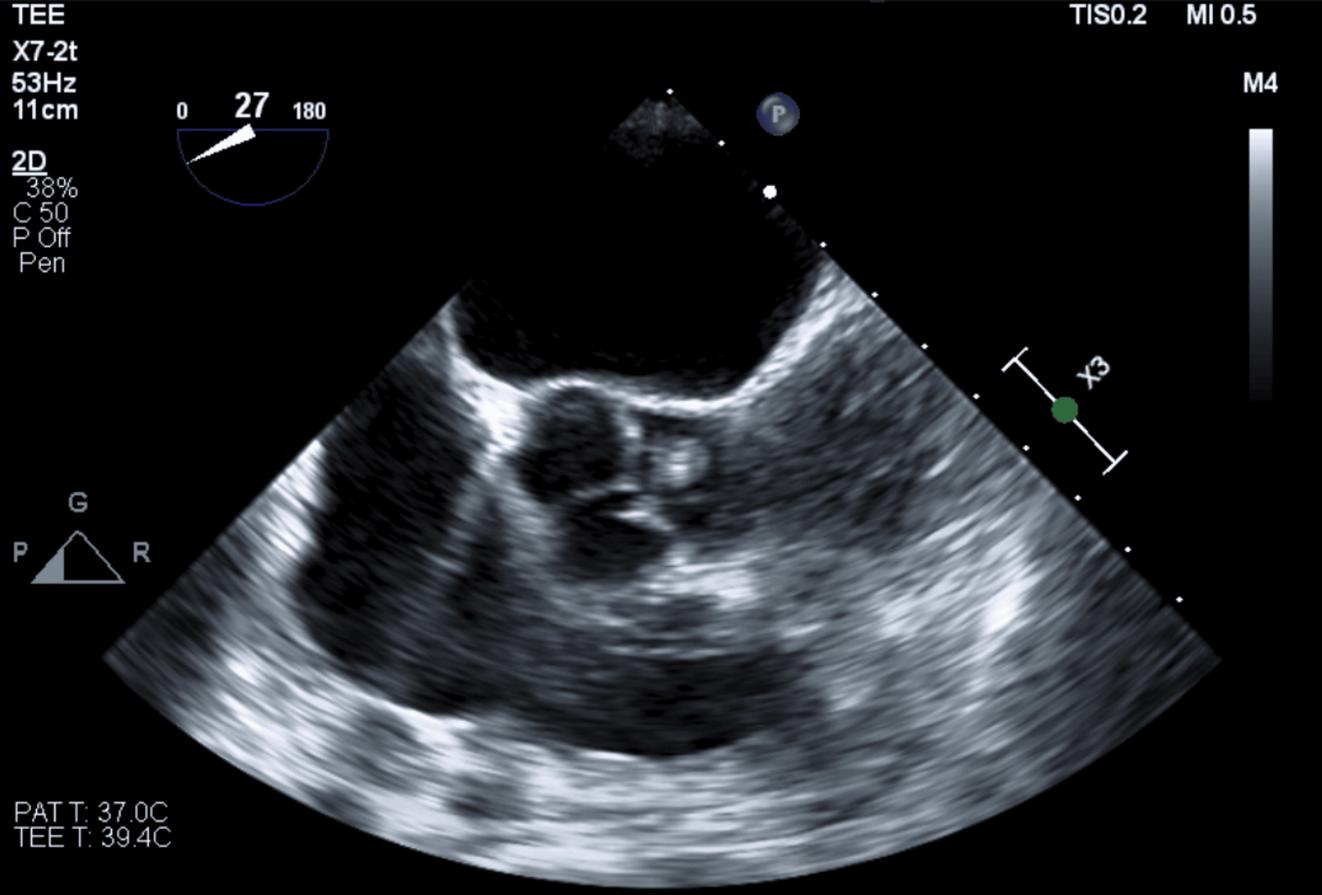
A transesophageal echocardiogram showing the mid-esophageal aortic valve short-axis view at approximately 27° A transesophageal echocardiogram shows a small, highly mobile, filamentous mass that is identified at the base of the noncoronary cusp
